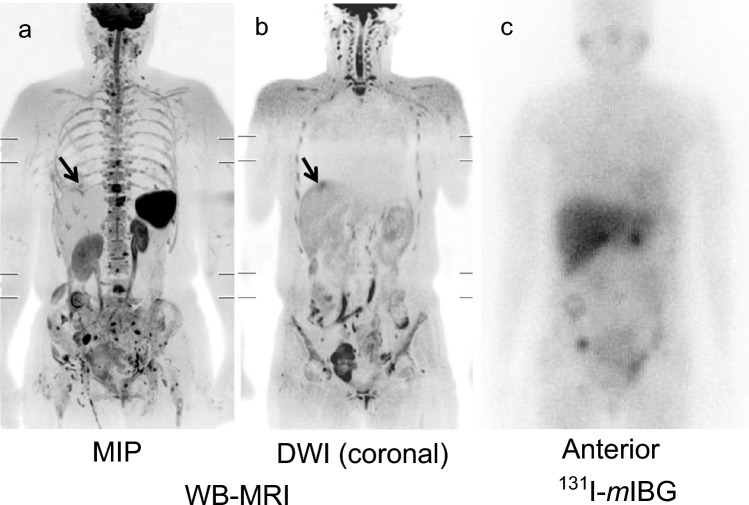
A case of liver metastasis that could only be detected by WB-MRI (patient No. 14). A female patient in her 40s with multiple metastases of paraganglioma (liver, vertebra, pelvis, peritoneum). Liver metastasis could only be detected by WB-MRI (arrows). Conversely, *m*IBG images could not detect metastasis due to physiologic liver accumulation. (a,b) The MIP of WB-MRI and DWI coronal image (WB-MRI). (c) Post-therapy ^131^I-*m*IBG scintigram (anterior). *WB-MRI* whole-body magnetic resonance image, *MIP* maximum intensity projection, *DWI* diffusion-weighted image, *mIBG meta*-iodo-benzylguanidine.
